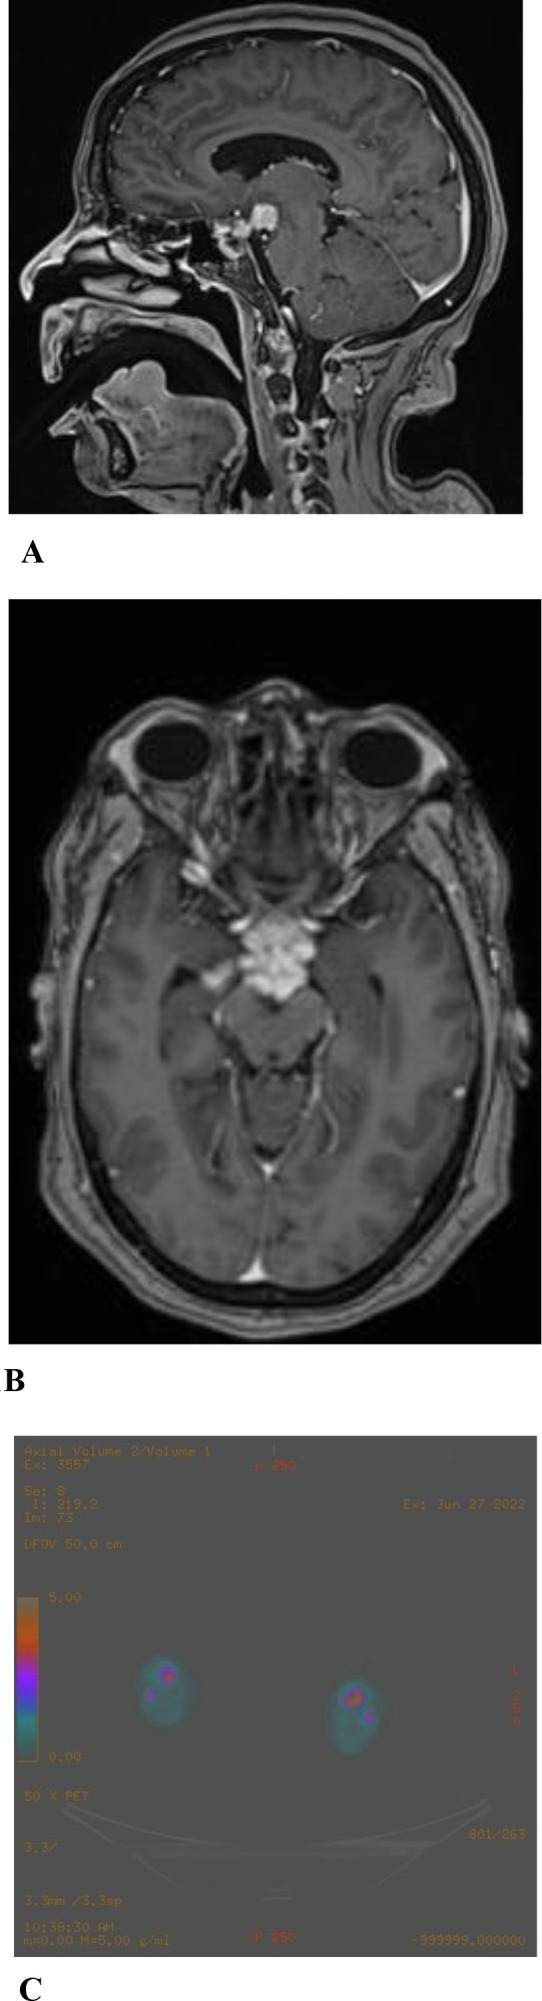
Pre-treatment Radiologic images (2022): Axial (B) and sagittal (A) post-contrast T1-weighted Brain MRI showing a lobulated enhancing suprasellar mass with infiltration of the pituitary stalk,tuber cinereum and hypothalamus, lamina terminalis and right mesial temporal lobe. Axial (C) FDG PET/CT showing bilateral tibial hypermetabolic heterogeneous uptake.
